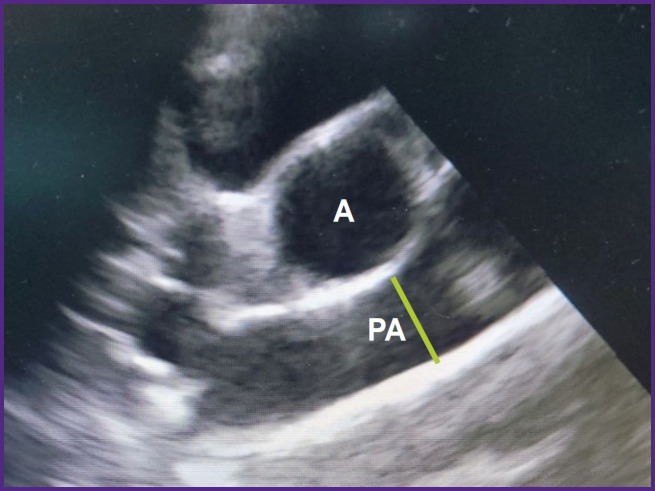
EchCG image of the pulmonary artery; right parasternal projection, shortaxis view A — aorta, PA — pulmonary artery
